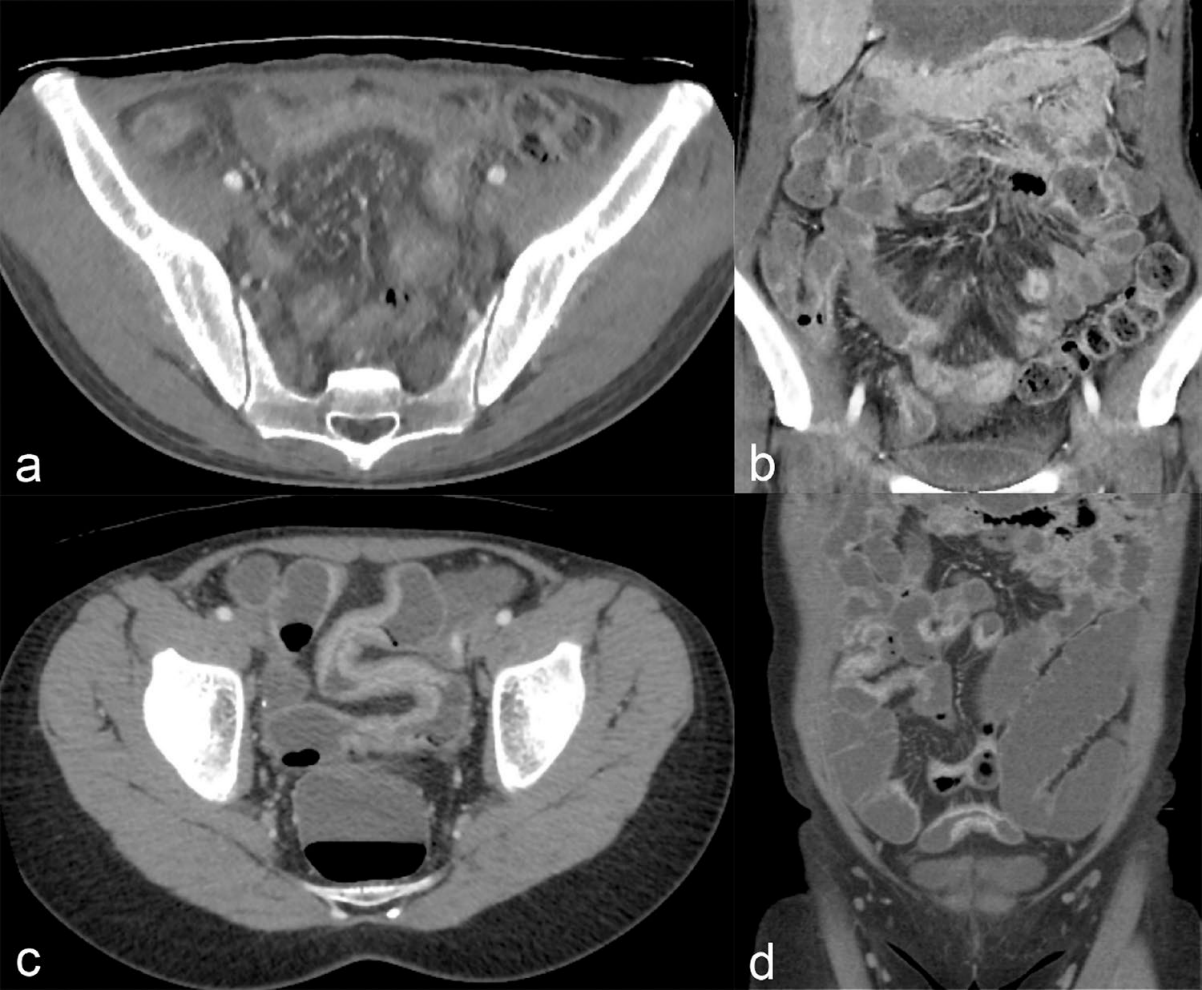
Representative images of (a) and (b) standard-dose CT enterography and (c and d) reduced-dose CT enterography in two patients. Both cases show active small bowel Crohn's disease, manifested with mural hyperenhancement, bowel wall thickening, and multiple skin lesions. In the subjective image quality assessment, (a, b, c), and (d) represent scores of 2/1, 3/3, 4/3, and 4/3 (reviewers 1/2), respectively.
